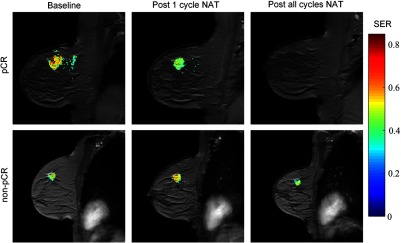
An example of semiquantitative DCE-MRI of a patient achieving pCR (top row) and a patient not achieving pCR (bottom row) before (first column), after the first cycle (second column), and at conclusion of all NAT (third column). The signal enhancement ratio (SER) overlaid on a high resolution anatomical MRI scan is shown. The high intensity regions-of-interest indicate gross tumor burden. The patient who ultimately achieved pCR demonstrates reduced tumor burden after one cycle of NAT.
